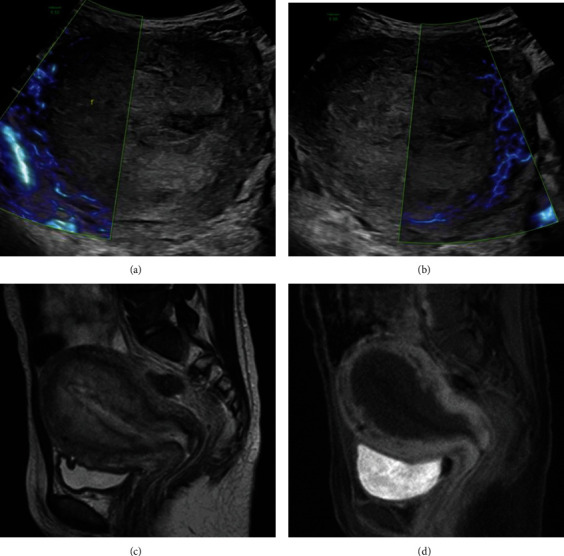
(a, b) Cross section of the internal myometrium in abdominal ultrasonography on postpartum day 24. Abdominal ultrasonography does not show blood flow in the internal myometrium by abdominal ultrasound. (c, d) MRI shows heterogeneous high intensity in internal myometrium on sagittal T2-weighted images (c) without contrast enhancement on sagittal postcontrast T1-weighted images (d), indicating necrosis.
